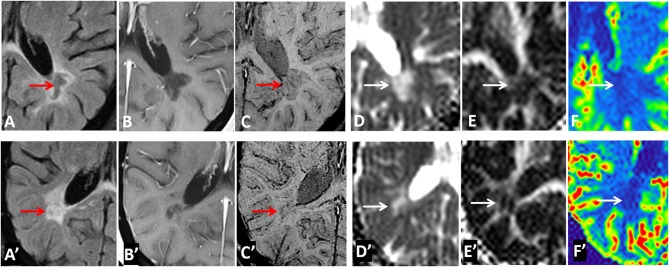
Representative images of Type-2 lesions in two MS patients (top row from a 43-year-old female patient and bottom row from a 37 female patient) include FLAIR (A,A′), Gd-enhanced T1-weighted (B,B′), and SWI (C,C′), as well as parametric maps of MD (D,D′), FA (E,E′), and CBF (F,F′) The slightly hypointense (top row) or isointense (bottom row) lesion on SWI (arrow) showed a remarkable increase in MD, and decrease in FA and CBF, suggesting the chronic necrotic lesions indicated by the hypointensity on FLAIR, and T1-weighted imaging (i.e., top row) have severe microstructural destruction and disturbed perfusion.
